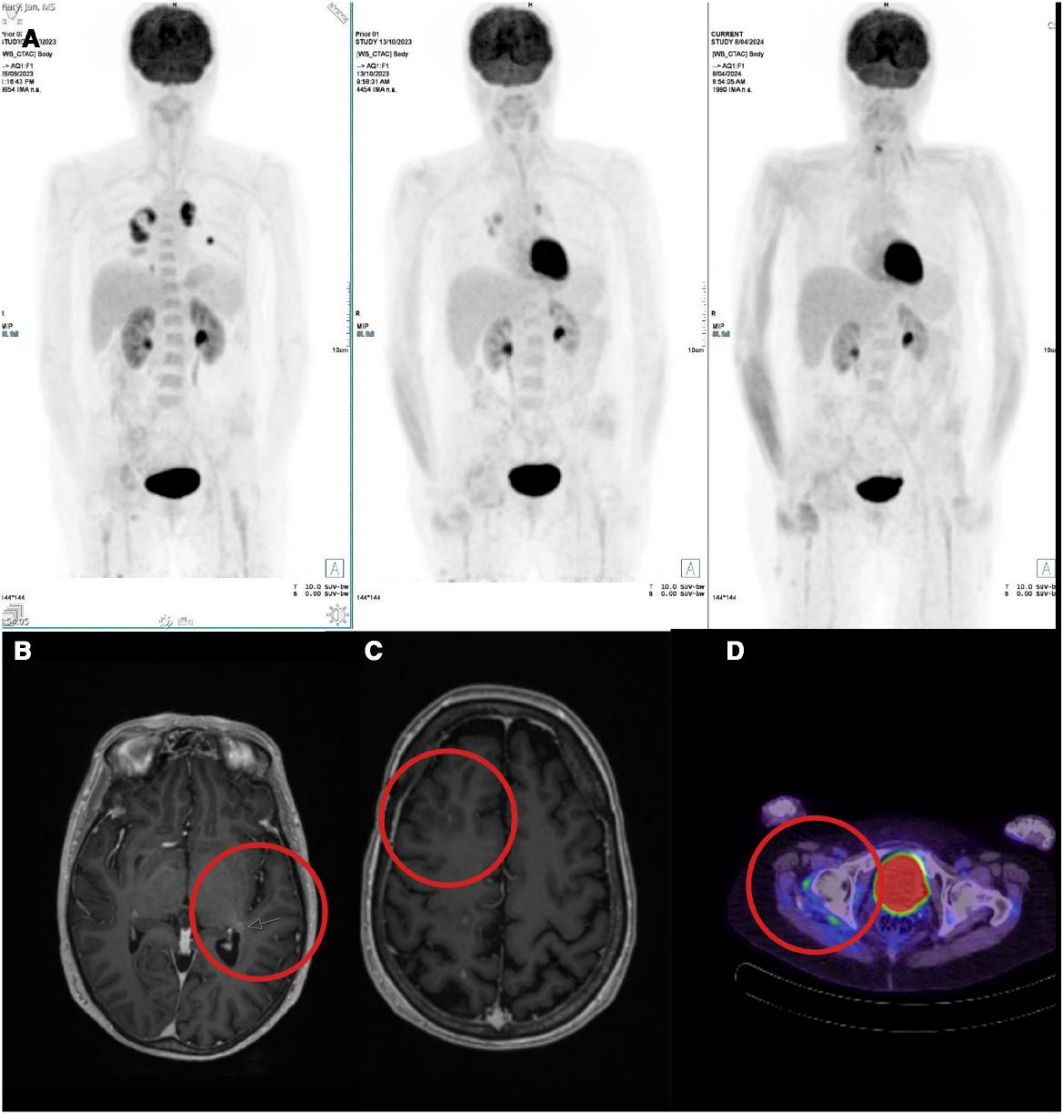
A, B, and C demonstrate areas of disease response with various imaging modalities in April 2024 after 10 months of treatment with nivolumab. A, highlights serial FDG-PET-CT scans through the course of treatment with nivolumab. The first panel on the left was conducted in June 2023 prior to commencing nivolumab. It demonstrated new large nodal and pulmonary metastases in the thorax. The right acetabular metastasis already had marked reduction in avidity, possibly secondary to radiotherapy. The middle panel demonstrates significant but incomplete disease response in the thoracic nodal and pulmonary metastases after 4 cycles of nivolumab (October 2023). The last panel on the right shows an FDG PET-CT scan performed in April 2024, which indicated further metabolic response with very mild metabolic activity in the aortopulmonary region, which may have been due to posttreatment inflammatory change (in view of the area of dense calcification). There was no evidence of metabolically active disease elsewhere. B, MRI scan demonstrates the significant reduction in the lesion in the left retrolentiform region which measured 30 × 23 mm in Fig. 3A. C, MRI scan demonstrates the disease response in the lesion located in right middle frontal gyrus which measured 16 × 14 mm in Fig. 3A. D, demonstrates disease response in the right acetabulum compared to Fig. 3C.
